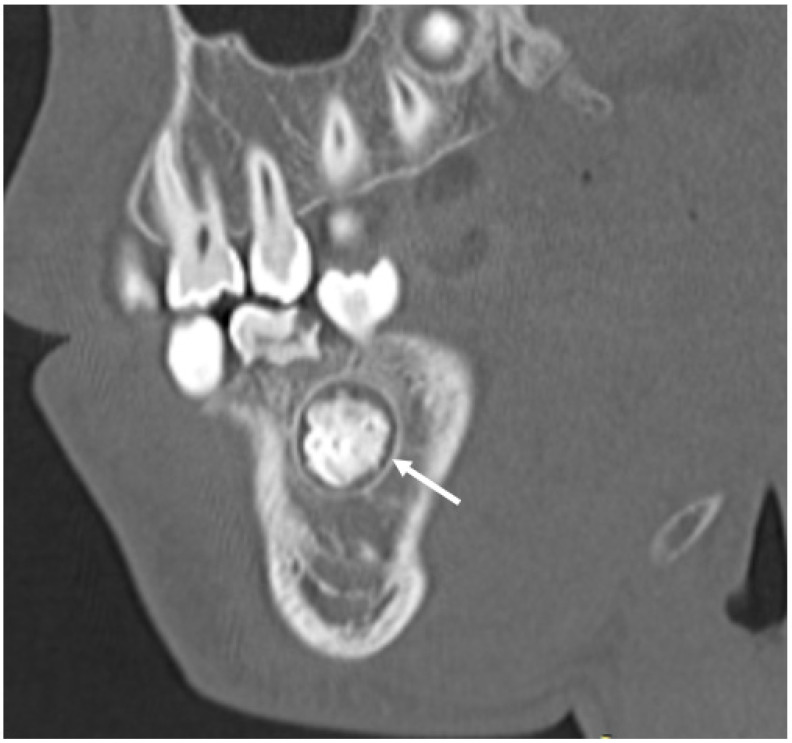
Sagittal section CT demonstrating heterogeneous mineralized tissues with evidence of bone and enamel densities. The uniform surrounding radiolucency (white arrow) suggests the presence of a periodontal ligament space compatible with a diagnosis of an odontoma.
